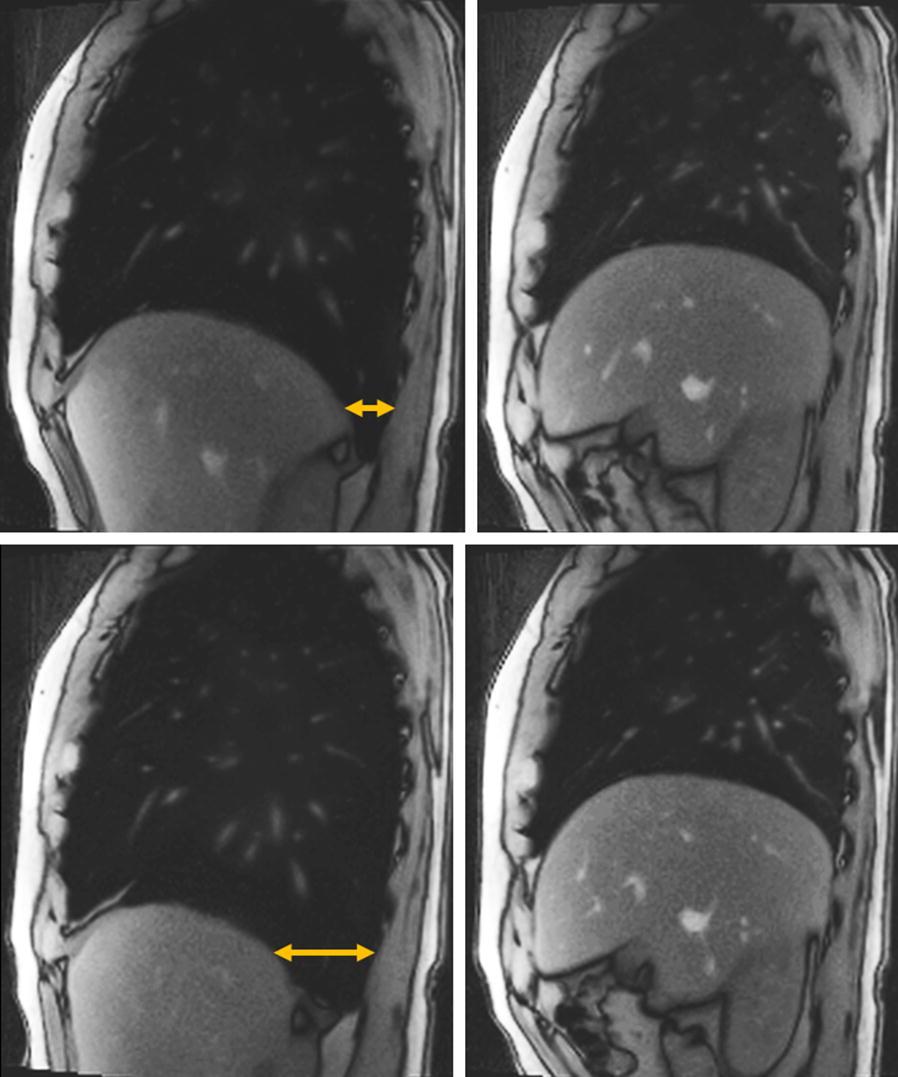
Real-time MRI of thoracic and abdominal breathing. Selected sagittal real-time images through the right dome of the diaphragm during thoracic and abdominal respiration. Upper left: thoracic breathing at deep inspiration and upper right: deep expiration. Lower left: abdominal breathing at deep inspiration and lower right: deep expiration. Inspiration causes elevation of ribcage, widening of anteroposterior thoracic diameters, contraction of the diaphragm with downward movement of its dome and enlargement of the intrathoracic volume. The corresponding widening of the costodiaphragmatic recess (arrows) is more pronounced during abdominal inspiration
